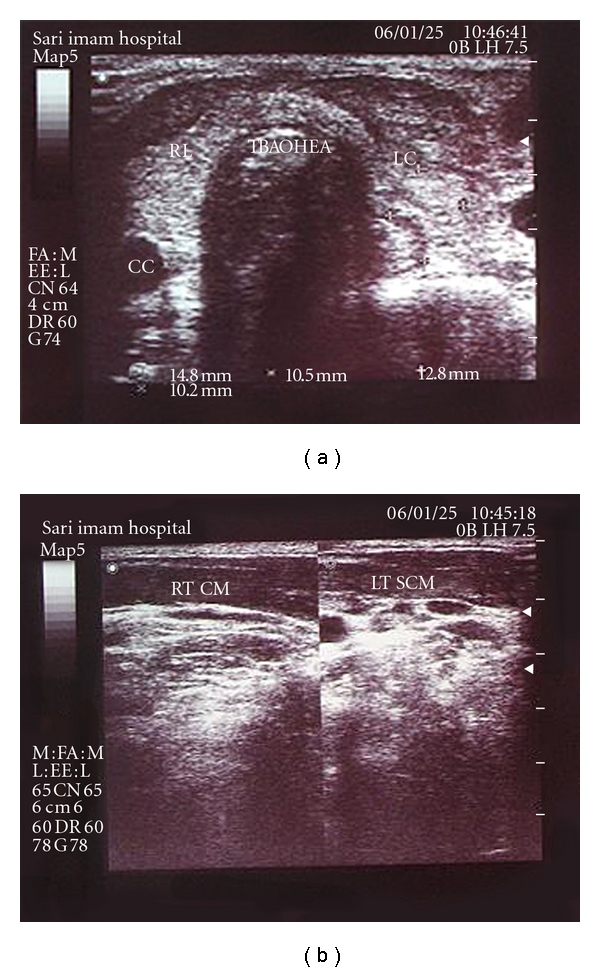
Second sonography findings in the second case.
